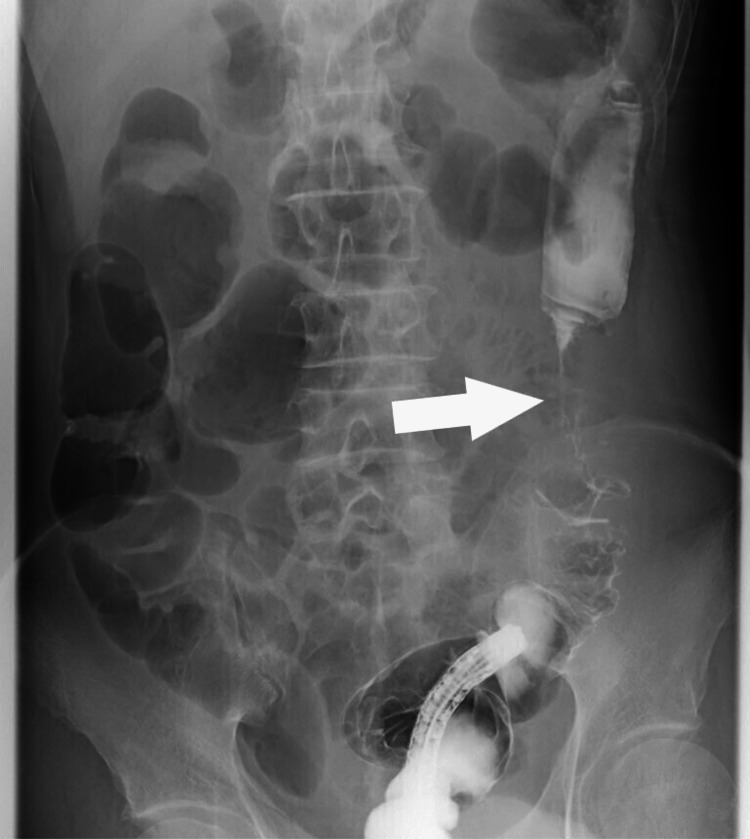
Abdominal X-ray A Gastrografin enema showed severe stenosis in the descending colon (white arrow).
